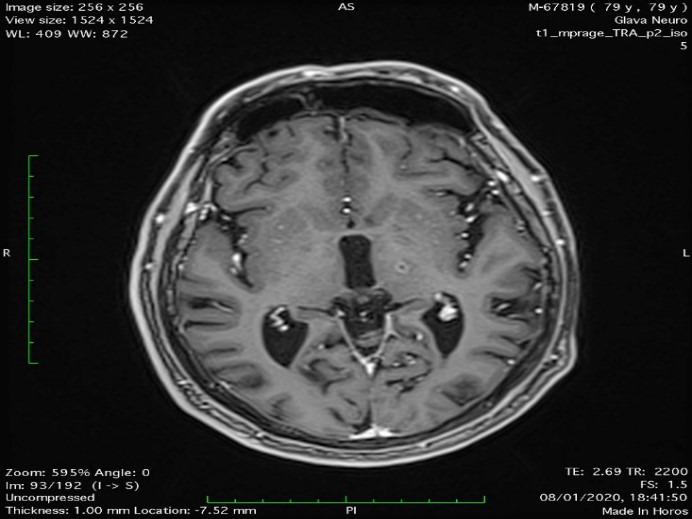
A brain magnetic resonance image (eight months after gamma knife thalamotomy) of case 1 shows an axial plane of a T1, magnetization-prepared rapid gradient echo sequence with adequate irradiation lesion of the left thalamic ventral intermediate nucleus.
